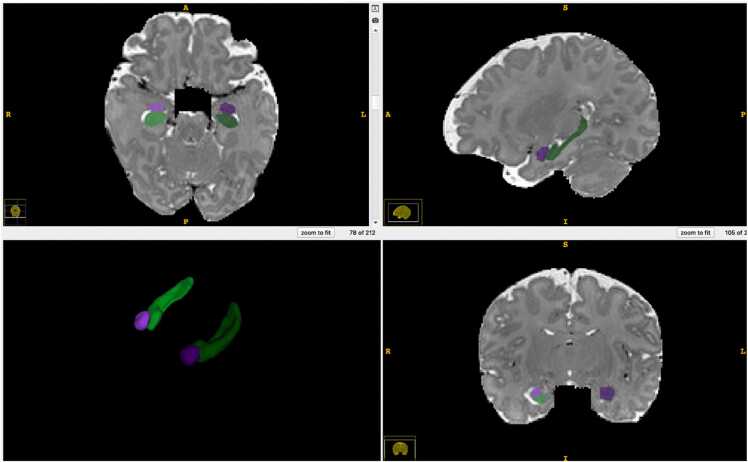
Regions of Interest for the Present Study Note. 3D visualization of representative example of hippocampus and amygdala segmentation on MRI T1 (T2) weighted scan. Purple = amygdala; Green = hippocampus; Left = darker color; Right = brighter color.
